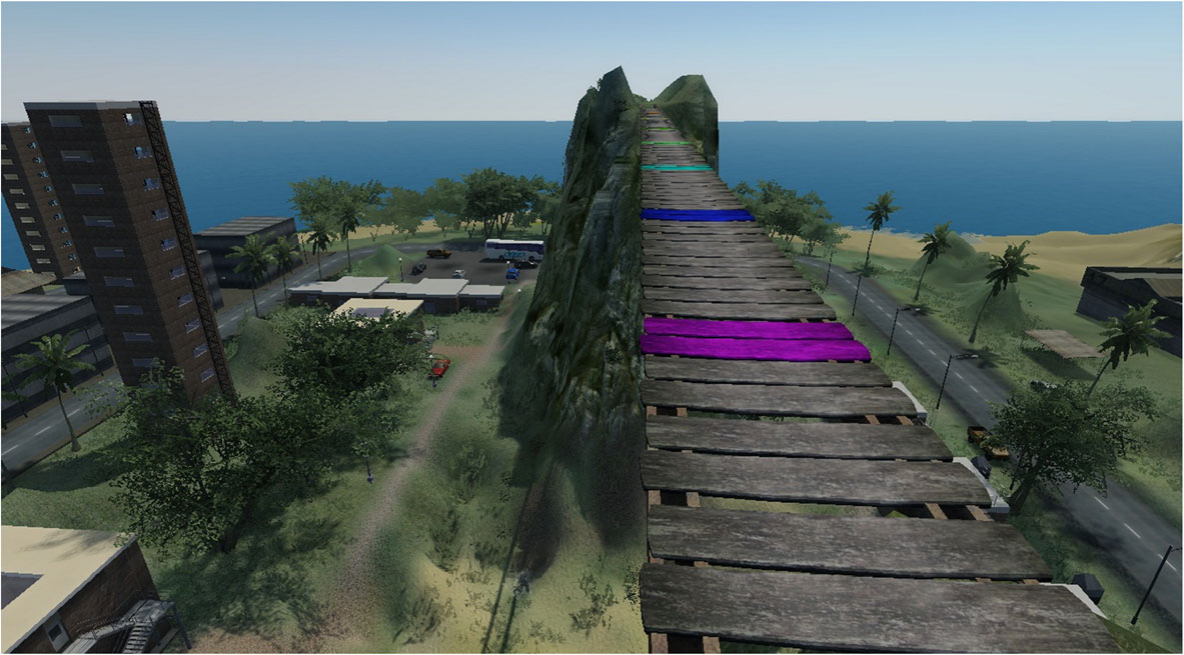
Screen capture of the virtual environment created for the ^18^F-FDG PET scan sensitized by virtual exposure consisting of a 50-m (165 ft) high wooden bridge devoid of any handrails and made of spaced planks. Coloured planks are dependent on the distance covered by the subject while immersed in this virtual environment for calculating the behavioural avoidance test (BAT) score on a 10-point scale
